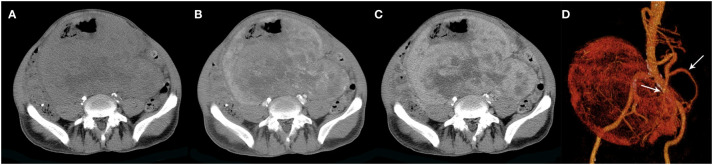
A 60-year-old man with SFT. (A) Precontrast CT scan shows a huge irregular mass with areas of necrosis in the pelvic cavity. (B) Numerous intratumoral vessels are seen in the arterial phase on post-contrast CT image. (C) Heterogeneous marked enhancement is detected in the venous phase. (D) Collateral feeding arteries arising from the inferior mesenteric artery are detected on 3D VR image (white arrows).
